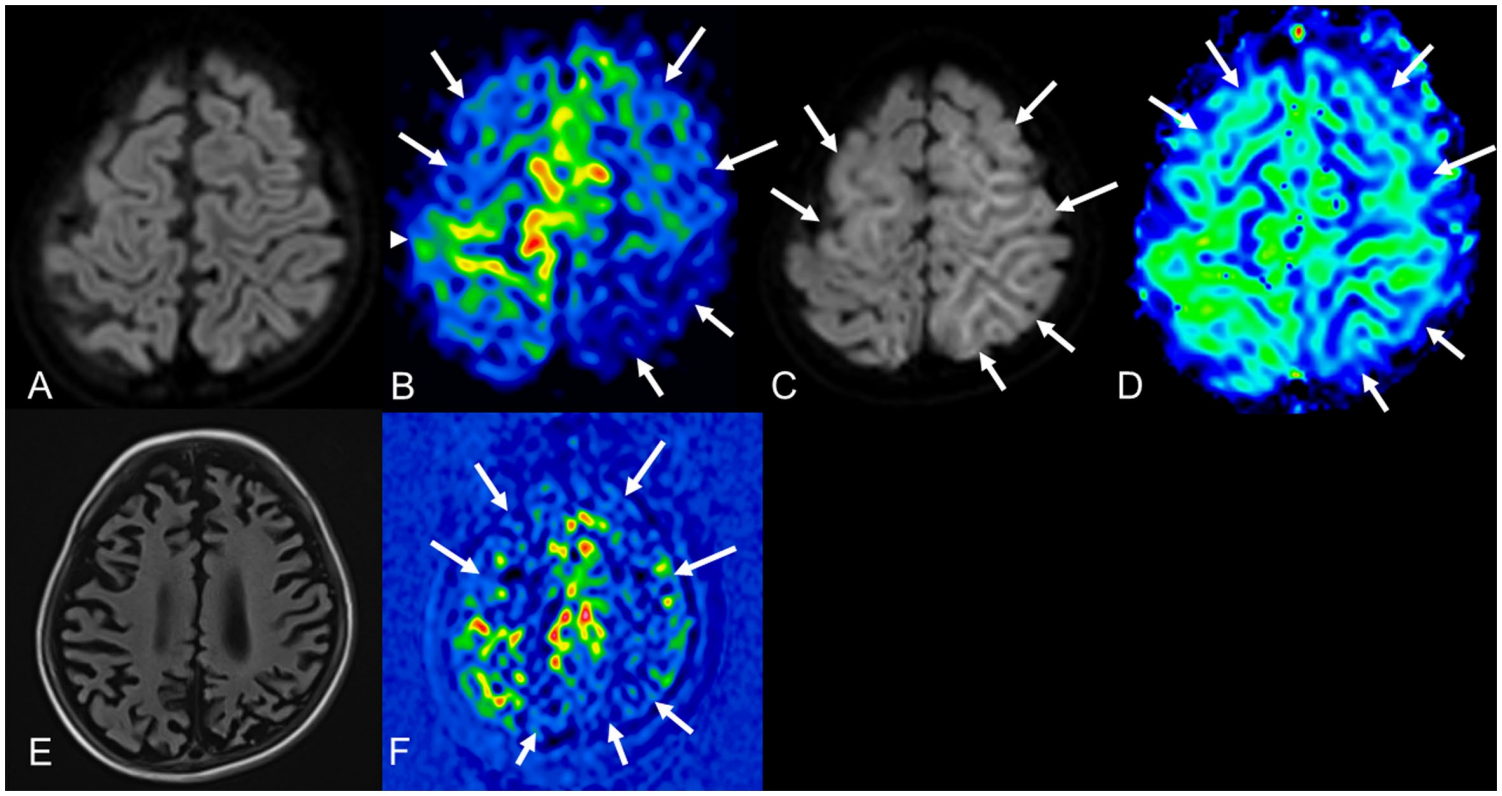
A 34-month-old AESD female with residual psychomotor retardation and epilepsy. DWI 8.5 h after early seizure (A) shows normal, but pulsed ASL (B) shows severe hypoperfusion (arrows) in the bilateral frontal and left parietal cortex with central sparing (arrowhead). She underwent targeted temperature management under continuous sedation and ventilation, and late seizures did not occur. DWI 5 days after early seizure (C) shows hyperintensity in the bilateral frontal and left parietal cerebral subcortical white matter (BTA, arrows). The distribution of BTA is consistent with the area exhibiting hypoperfusion on ASL after early seizure. Pulsed ASL 5 days after early seizure (D) shows slight hypoperfusion (BTA, arrows). FLAIR imaging (E) and pulsed ASL (F) 6 months later showed severe atrophy and hypoperfusion in bilateral frontal and parietal lobes (arrows). AESD, acute encephalopathy with biphasic seizures and late reduced diffusion; DWI, diffusion-weighted imaging; ASL, arterial spin labeling; BTA, bright tree appearance; FLAIR, fluid-attenuated inversion recovery.
